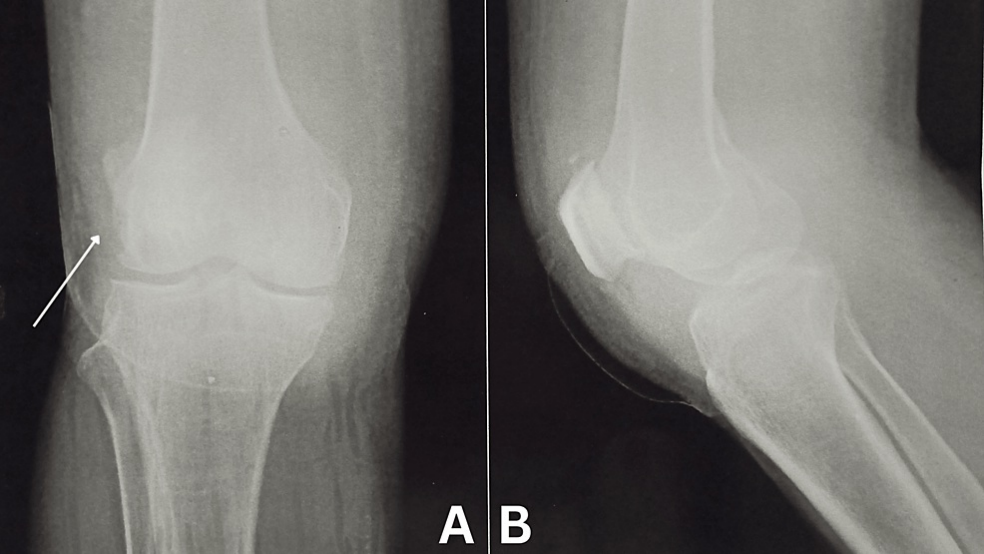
X-Ray of right knee (AP and lateral views) A) AP view; B) Lateral view The arrow points to the sclerotic changes of lateral condyle of femur. AP: Antero-posterior
